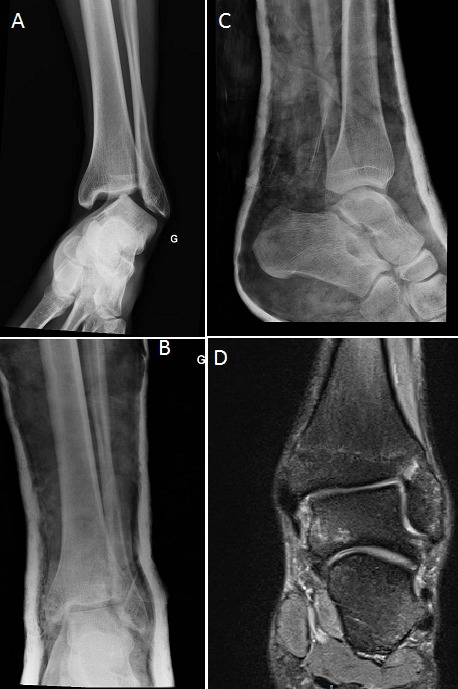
A) radiographie de la cheville gauche montrant la luxation; B) radiographie de la cheville de face après réduction; C) radiographie de la cheville de profil après réduction; D) IRM de la cheville de contrôle montrant intégrité des ligaments péri-articulaire
